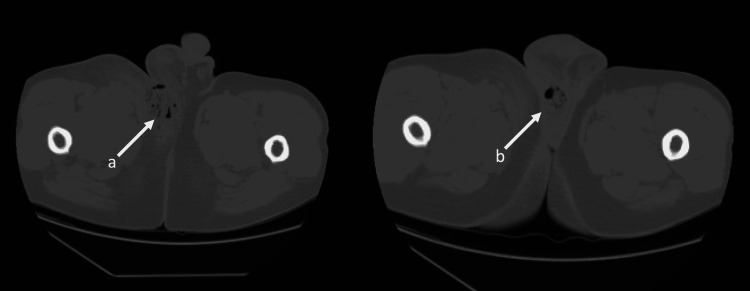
CT scan of the abdomen and pelvis The image shows marked inflammatory stranding and foci of air within the right groin, extending into the medial proximal right thigh and right scrotal wall (a). There was more localized fluid and air in the posterior right hemiscrotal wall (b) CT: computed tomography
